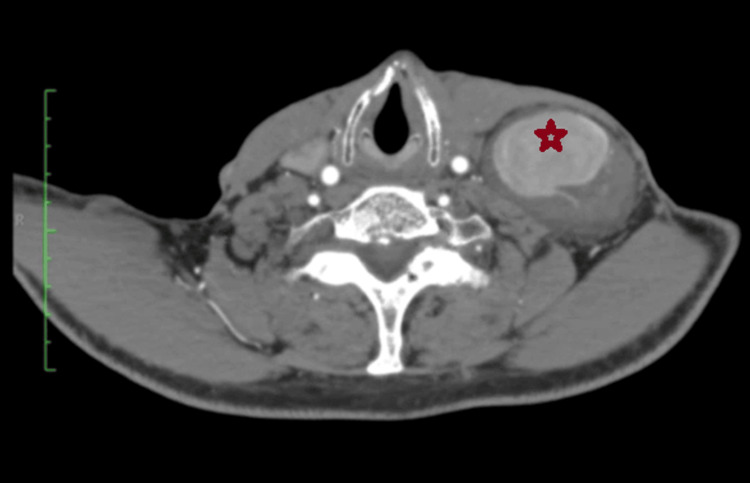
CTA of the chest showing a large, left thyrocervical trunk pseudoaneurysm (at the root) with associated mural thrombus (red star). CTA: computed tomography angiogram
